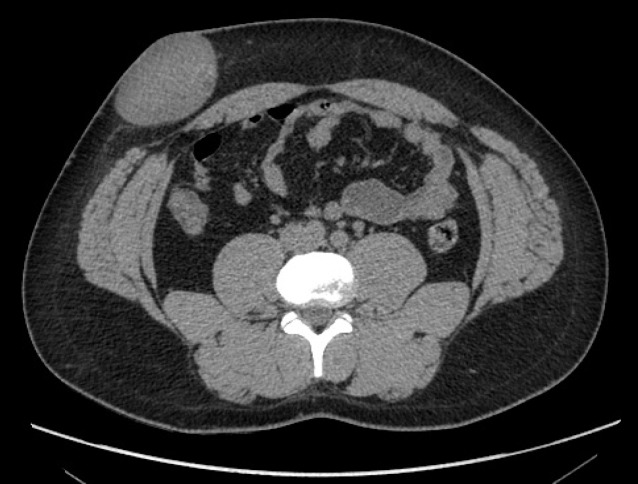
Basal abdominal CT scan revealing an oval-shaped, homogenous mass, with muscular density, developed in the subcutaneous tissues of the anterior abdominal wall. The mass is well-circumscribed with regular limits clearly differentiated from the surrounding structures
